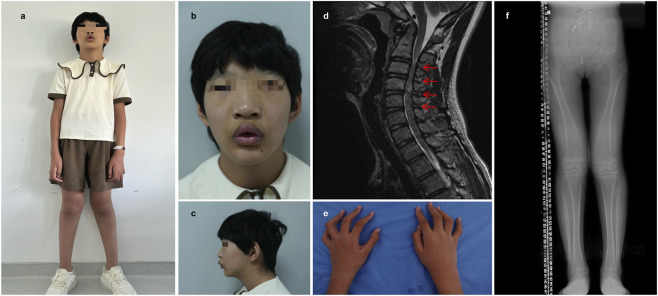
Clinical and radiological features of the patient. (a) Full-body photograph showing generalized overgrowth and genu valgum. (b,c) Frontal and lateral facial views showing characteristic facial features, including hypertelorism, a broad nasal bridge, large ears, and thick lips. (d) Sagittal cervical spine T2-weighted MRI showing cervical spinal canal stenosis; the AP diameter at the narrowest point was approximately 3 mm, and red arrows indicate cervical cord edema. (e) Photograph of both hands showing enlarged hands, elongated fingers, and flexion deformities of the distal interphalangeal joints. (f) Full-length standing radiograph of both lower limbs showing genu valgum. The hip-knee-ankle angle measured 4.8° on the right side and 3.7° on the left side.
